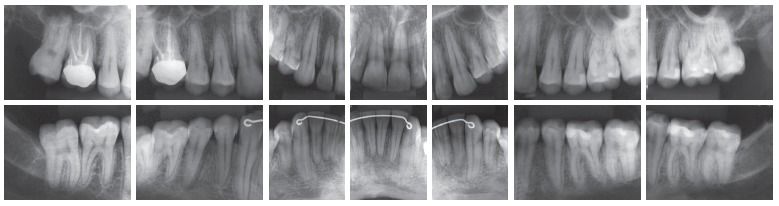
Final periapical radiographs.
